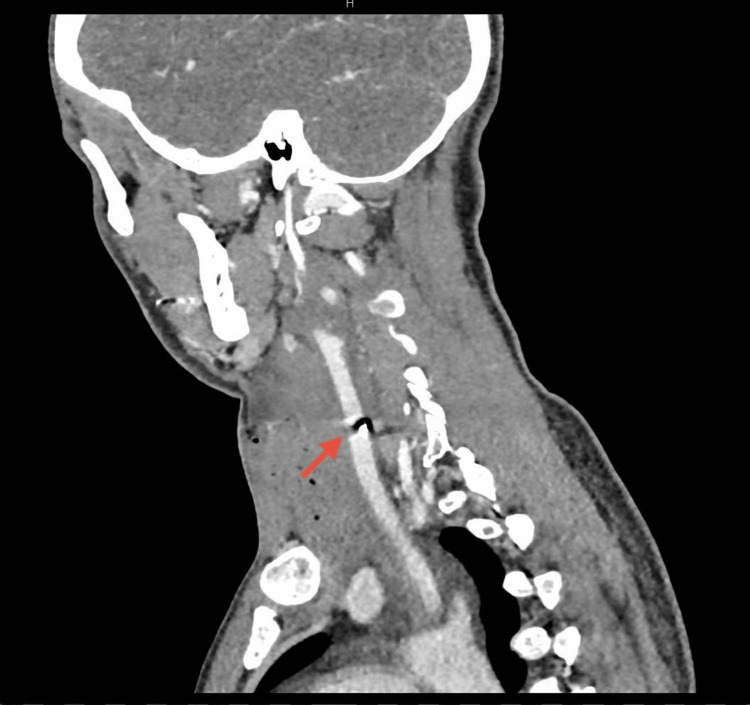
Preoperative CT. Left neck swelling showing air foci extending from the upper chest to the insertion of the left sternomastoid muscle, measuring about 7 x 10 cm, and associated with shifting of the trachea to the right, suspicious for a muscle tear. A left metallic foreign body with metallic artefact was noted very close to and abutting the common carotid artery.
